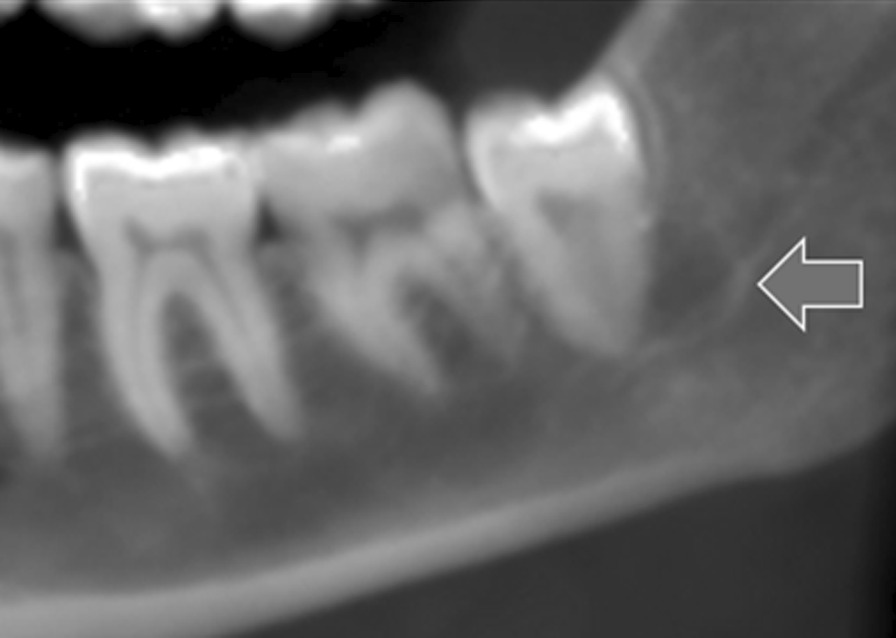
JAR associated with a lower third molar on reconstructed panoramic of CBCT images
